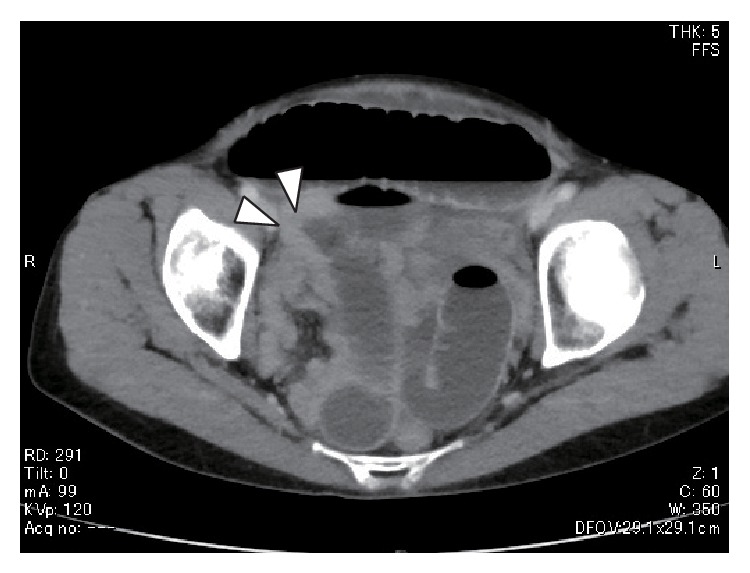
An abdominal computed tomography showed a small bowel dilatation with a caliber change (arrowhead).
